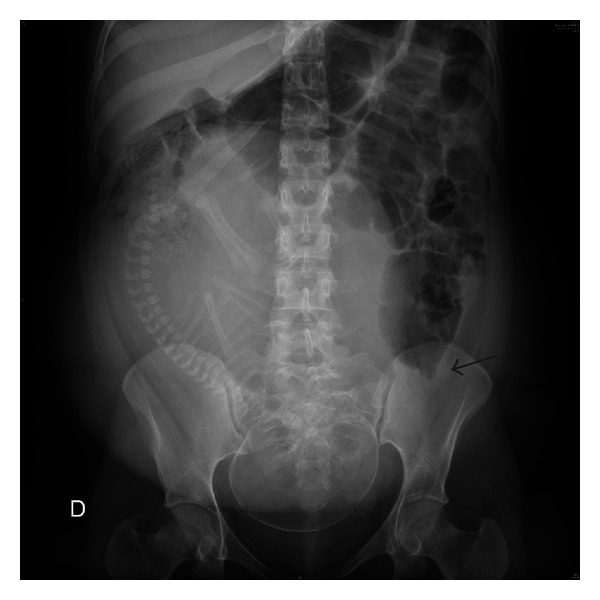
Abdominal X-ray showing dilatation of the small and large bowel loops. The arrow shows the stop at colon level. Displacement of the gravid uterus to the right is clearly seen.
